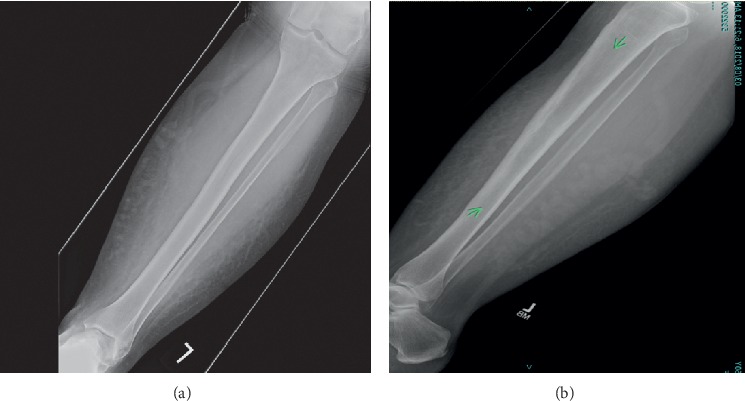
X-ray of the tibia fibula. (a) Antero-posterior (AP) view. (b) lateral view.
